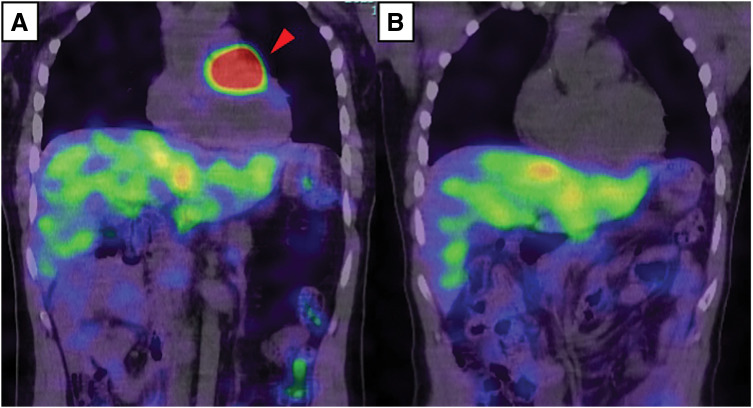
^123^I-MIBG SPECT/CT before and after surgery. (A) Preoperative fused coronal ^123^I-MIBG SPECT/CT demonstrates intense radiotracer uptake corresponding to the cardiac mass (arrowhead). (B) Postoperative fused coronal ^123^I-MIBG SPECT/CT shows complete resolution of abnormal uptake at the cardiac operative site. MIBG, metaiodobenzylguanidine; SPECT, single photon emission CT
